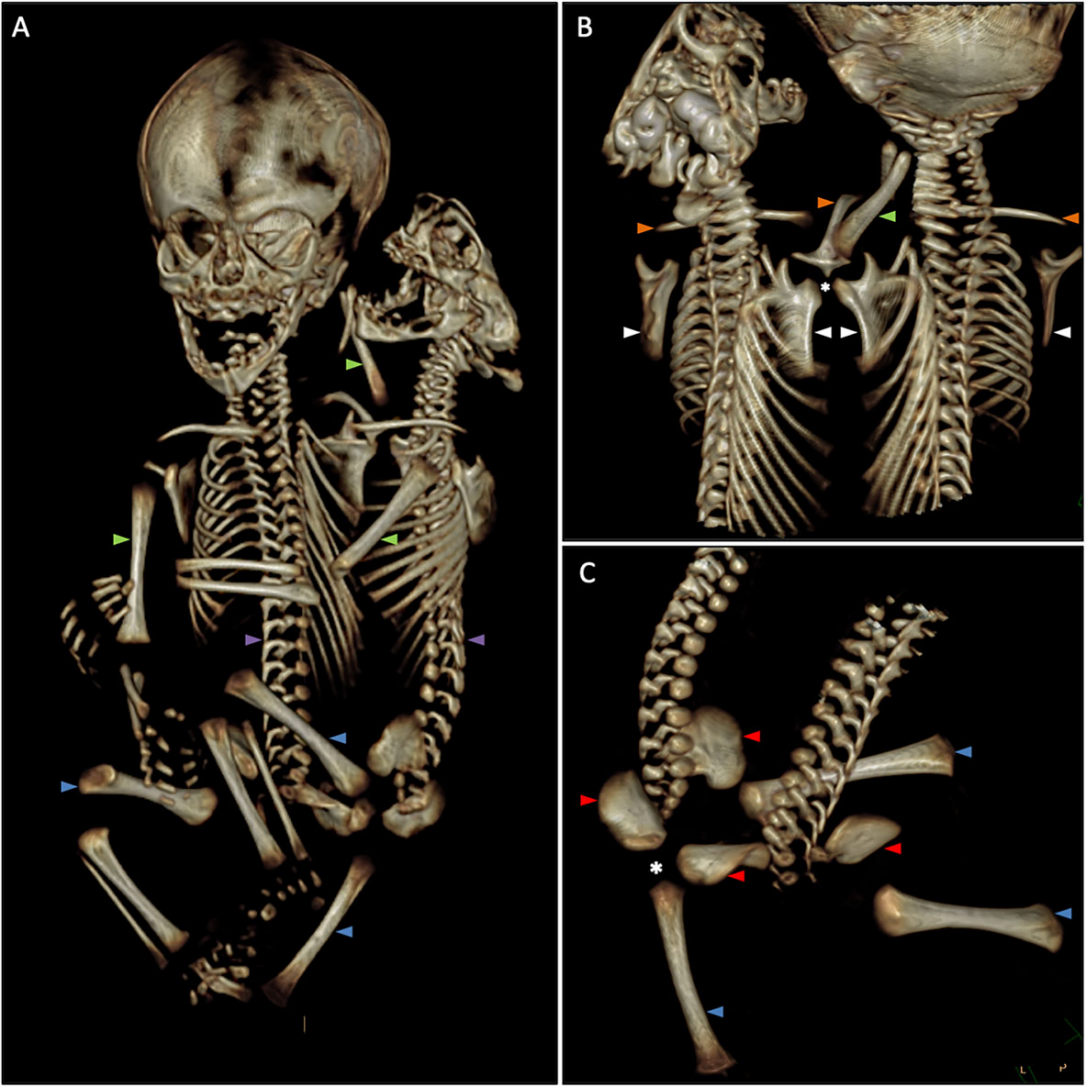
Three-dimensional computed tomographic reconstruction of the skeleton. a Overview showing normally developed right twin’s cranium and anencephaly in the left twin. Two separate vertebral columns (*purple arrow*) and three upper (*green arrow*) and three lower extremities (*blue arrow*) can be distinguished. b Dorsal view showing details of the third upper extremity (*green arrow*) articulating with a joint (*) formed by the right scapula of the left twin and the left scapula of the right twin (scapulae indicated with *white arrows*). Three clavicular bones can be distinguished (*orange arrow*). c Oblique, lateral detail of iliac bones (*red arrow*) and lower extremities (*blue arrow*). The third middle extremity is articulating with a joint (*) composed of the right iliac bone of the left twin and the left iliac bone of the right twin
